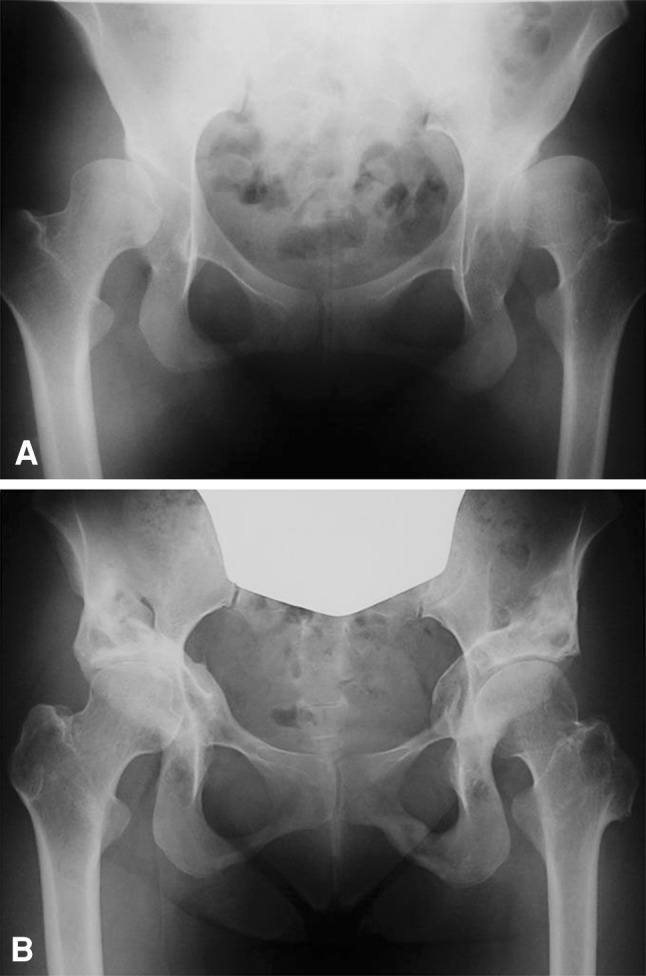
(A) A preoperative AP radiograph of the hips of a 19-year-old girl (the same patient whose radiograph is shown in Fig. 5) shows early-stage dysplastic osteoarthritis for the right hip and advanced-stage for the left hip. The right and left lateral CE angles are −13° and −44°, respectively. (B) Five years after right RAO and 7 years after left RAO, the right and left lateral CE angles are 33° and 20°, respectively. Both femoral heads were shifted medially and distally, and the left greater trochanter was pulled down and reattached. There was no progression of osteoarthritis.
